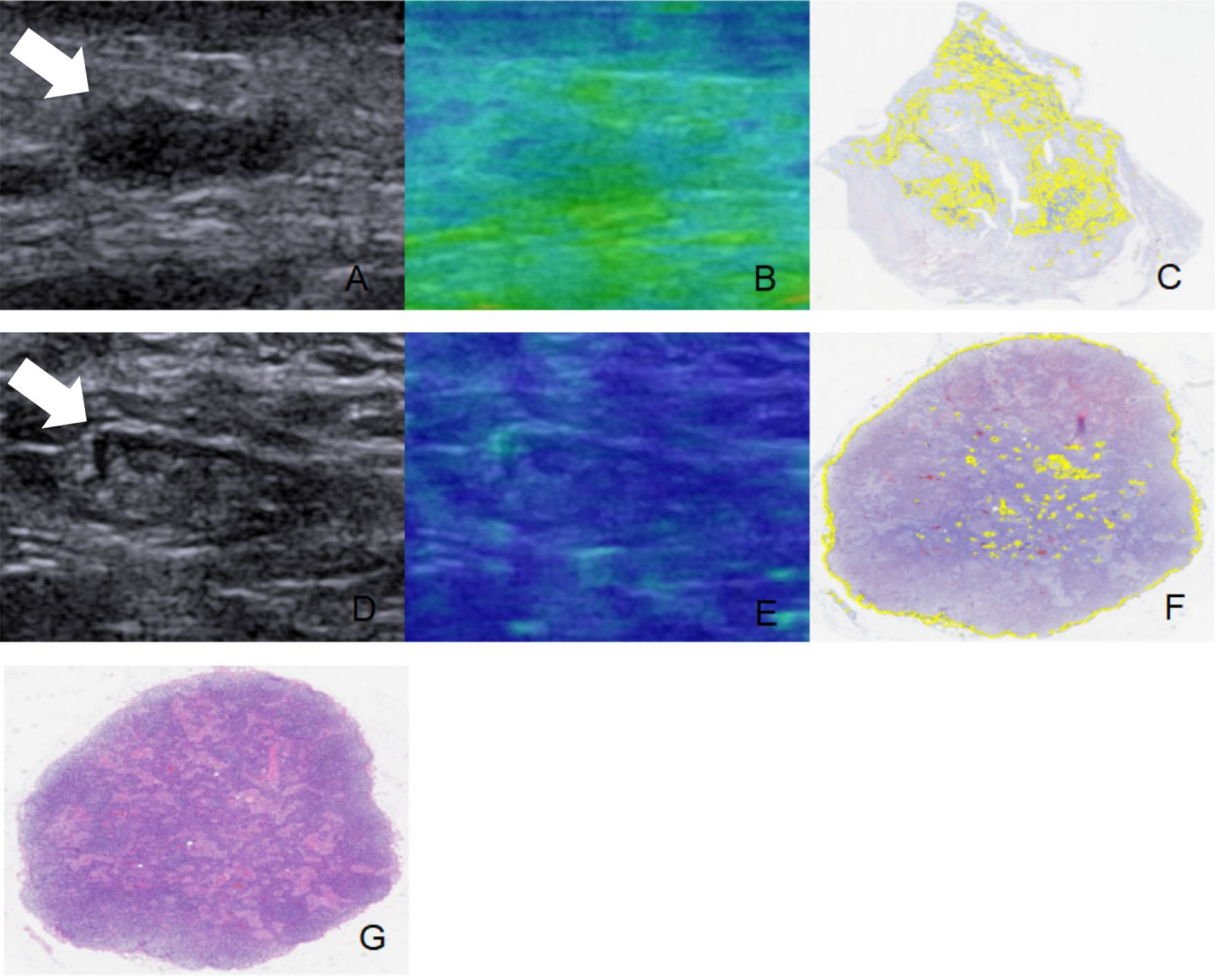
A breast cancer case with axillary pCR after NAC. A 32 year-old female patient with HER2 positive breast cancer had a residual breast lesion (arrow) with the size of 12 × 4 mm (A). The SWV of the breast lesion was measured to be 2.21 m/s, which was lower than the threshold value of 2.47 m/s (B). In addition, the CVF of the breast lesion was found to be 17.44%, which was lower than threshold value of 37.67% (C). For the LN (arrow) that was examined by US following NAC, it had a size of 11 × 4 mm, an oval shape, and a cortical thickness of 1.1 mm (D). The SWV of the LN was measured to be 1.33 m/s (E), and the CVF was 3.7% (F). A total of 16 axillary LN were resected after NAC, and no metastasis was found in all LNs (G). pCR, pathological complete response; NAC, neoadjuvant chemotherapy; HER2, human epidermal growth factor receptor2; SWV, shear wave velocity; CVF, collagen volume fraction; LN, lymph node; US, ultrasound
